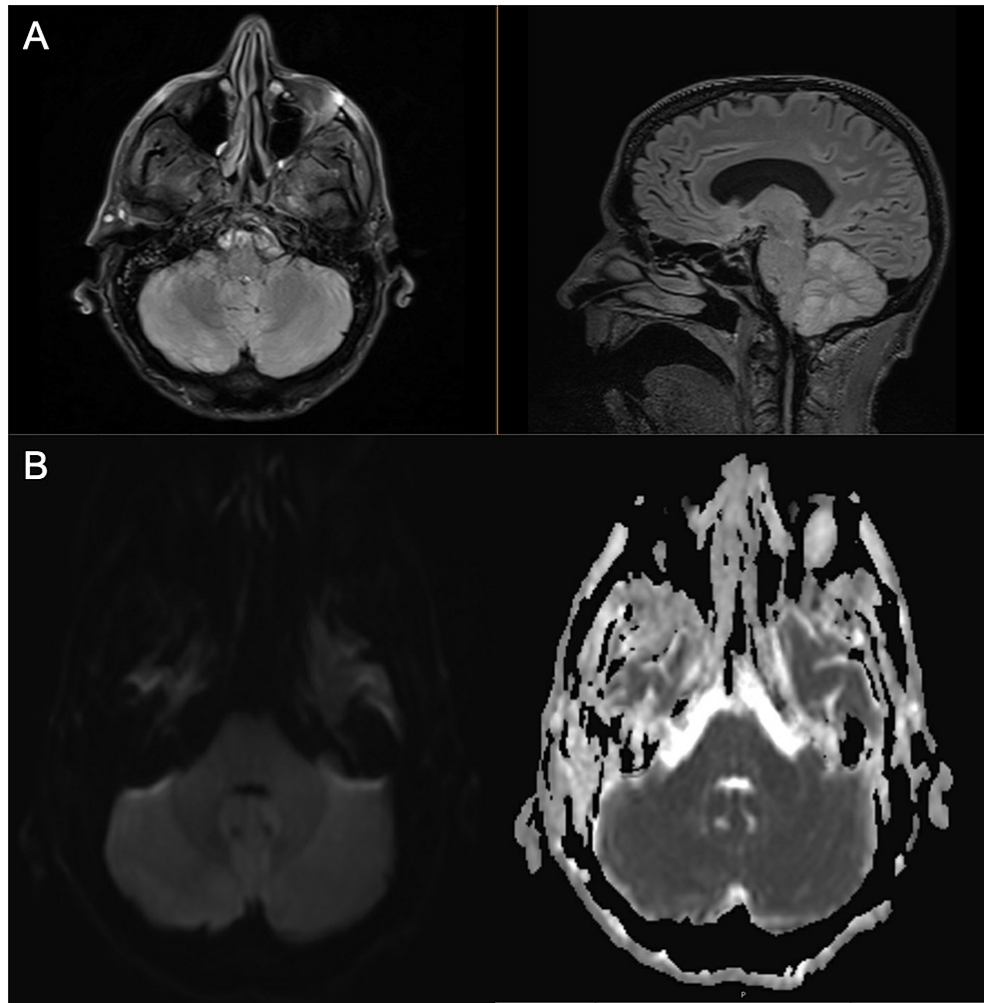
Follow-up MRI of the brain. A) MRI of the brain showing evidence of cerebellar FLAIR enhancement, consistent with cerebellitis B) DWI image with corresponding ADC map showing mild restricted diffusion. ADC: Apparent diffusion coefficient; DWI: Diffusion-weighted imaging; FLAIR: Fluid-attenuated inversion recovery.
